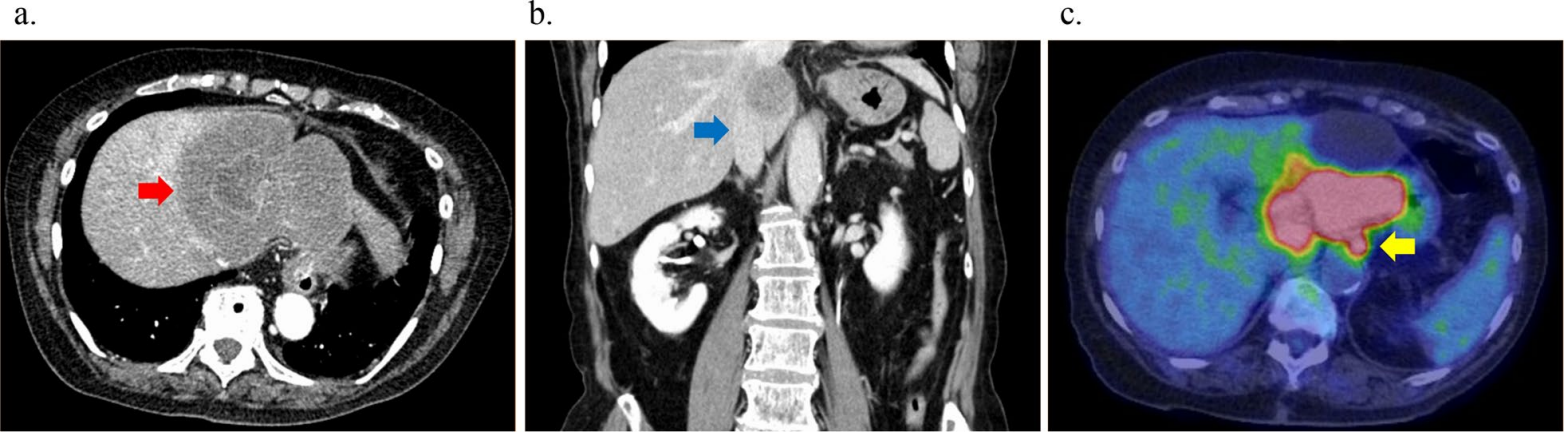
Radiological examinations before neoadjuvant chemotherapy (NAC). a, b Computed tomography (CT) shows a 100-mm tumor in the left hepatic lobe and segment 1. The tumor has invaded to the inferior vena cava (IVC) (a axial view of CT; b Coronal view of CT). c Positron emission tomography (PET) shows fluorodeoxyglucose (FDG) uptake around the lesser curvature nodes
